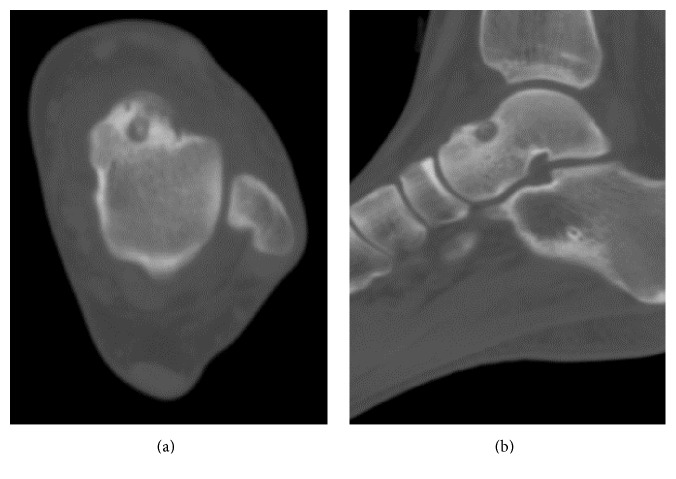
Axial (a) and sagittal (b) computed tomography images of the left ankle showing central calcification within a radiolucent lesion of the talar neck.
